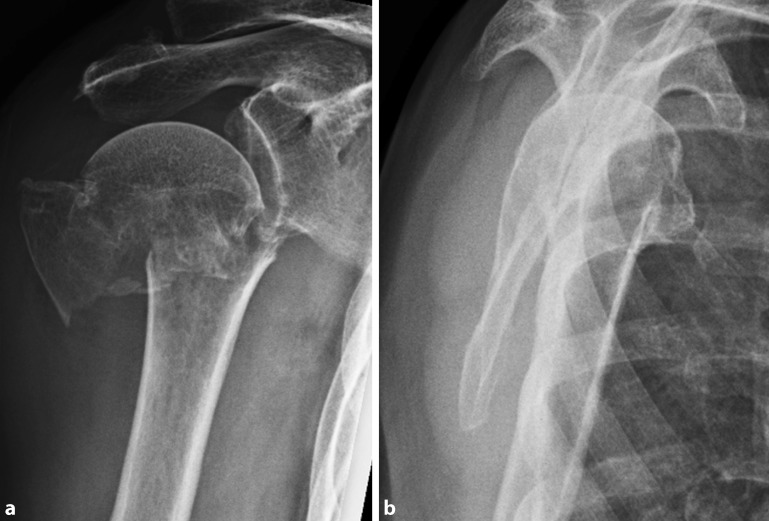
Preoperative radiographs of an 87-year-old female patient with valgus-displaced three-part proximal humeral fracture (a anteroposterior, b Y view)
